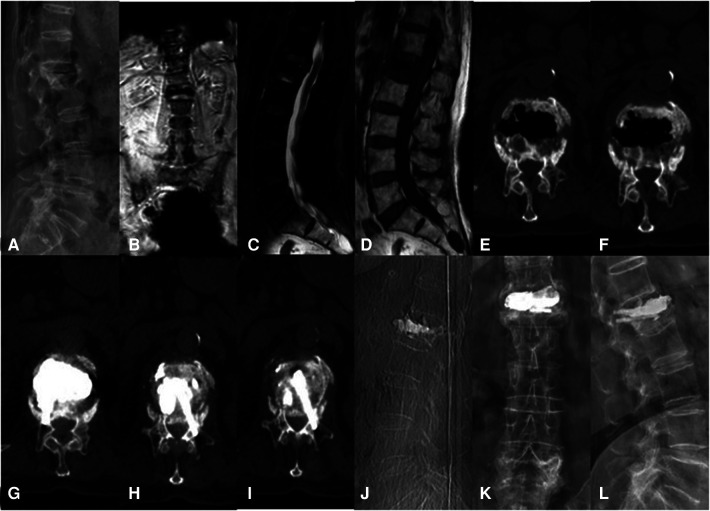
A 76-year-old woman with KD at L1 treated using the bilateral pedicle anchoring technique with PVP: (A) preoperative radiographic images; (B,C) coronal and sagittal T2-weighted MR image showing low signal intensity at L1; (D) the sagittal T1-weighted MR image showing low signal intensity at the same location; (E,F) CT scans showing the intravertebral vacuum sign; (G–J) CT scans after operation showing the bone-cement-filled cleft and vertebrae; bone cement dispersion was observed in both pedicles; (J–L) x-ray at 14 months after operation showing no delayed displacement of bone cement or adjacent vertebral fracture. KD, Kümmell's disease; PVP, percutaneous vertebroplasty.
